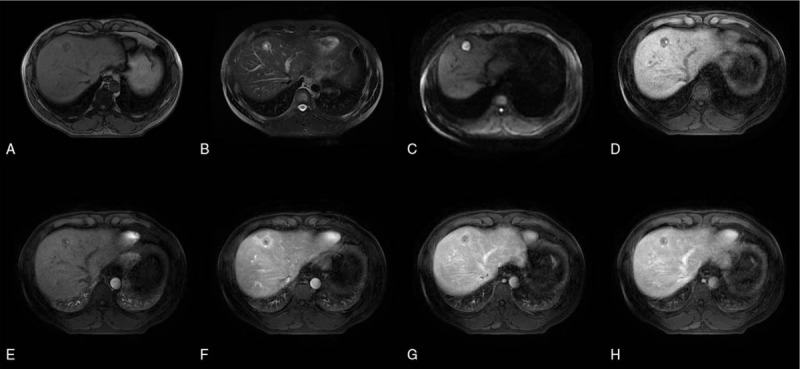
MRI was performed at initial admission. A 50-year-old male diagnosed as PHNET, grade G2. (A) Hybrid hypointense was observed on T1WI, and pseudocapsule could be seen. (B) Axis T2WI showed mixed high signal. (C) DWI image, (D) Enhanced anterior mask. (E) Early enhancement of arteries. (F) Late enhancement of arteries. (G) Enhanced venous phase. (H) Enhancement delay period.
